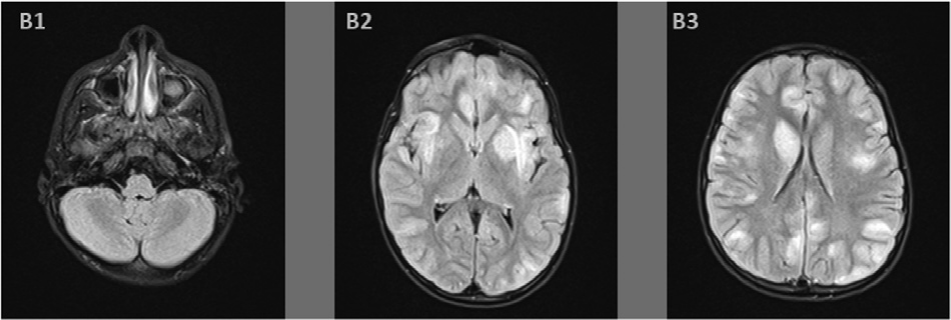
Fluid attenuated inversion recovery (FLAIR) sequence, the axial supratentorial images (B2, B3) show increased signal abnormalities, with nodular shape and poorly defined borders, involving bilateral central white matter, cortical gray matter, and basal ganglia. Infatentorial image (B1) show normal intense signal of cerebellar and mesencephalon parenchyma, with normal fourth ventricle caliber.
